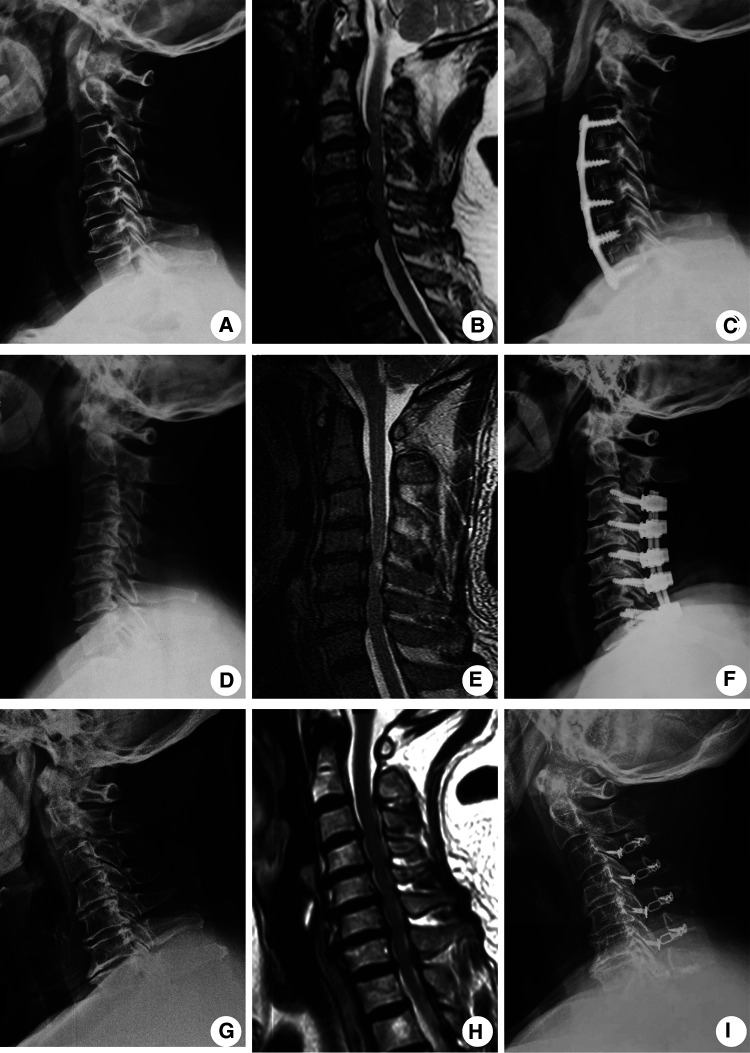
Typical radiological images showing four-level CSM patients treated with either ACDF, LAMP, or the LF approach. Representative preoperative lateral position x-ray radiograph (A), preoperative MRI image (B), and x-ray radiographs at a 2-year follow-up (C) of ACDF-treated patients. Representative preoperative lateral position x-ray radiograph (D), preoperative MRI image (E), and x-ray radiographs at a 2-year follow-up (F) of LF-treated patients. Representative preoperative lateral position x-ray radiograph (G), preoperative MRI image (H), and x-ray radiographs at a 2-year follow-up (I) of LAMP-treated patients.
